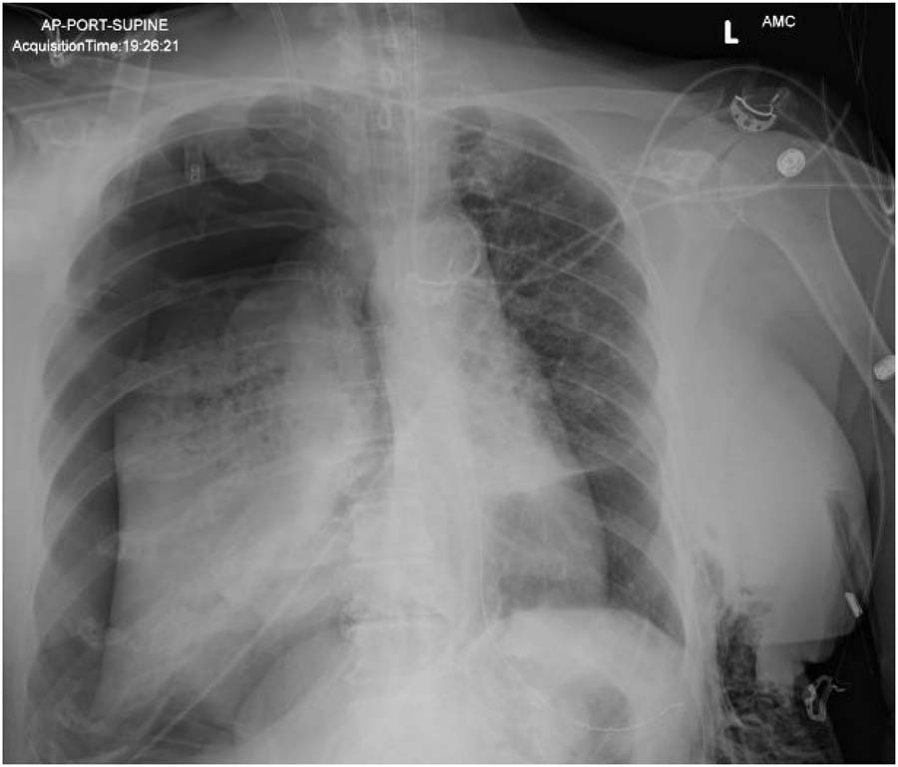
AP supine chest radiograph showing right-sided pneumothorax.
